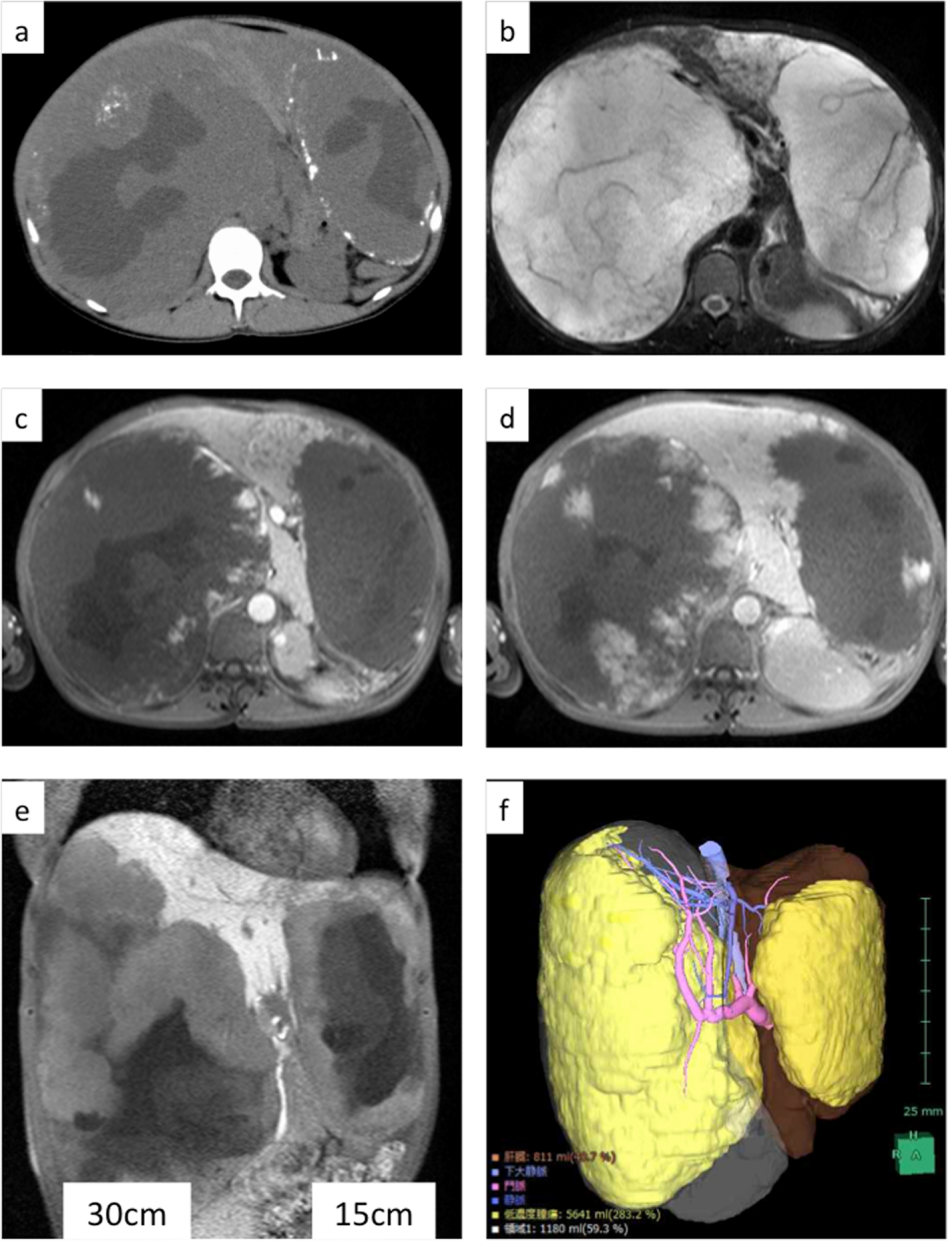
Plain computed tomography (CT) showed giant liver hemangiomas with lipiodol deposits (a). Magnetic resonance imaging (MRI) showed a 30-cm liver hemangioma in the right lobe and 15-cm liver hemangioma in the left lateral section (b T2 image, c arterial phase, d portal phase, e hepatobiliary image). There is normal liver parenchyma with tiny hemangioma in segment 4. According to 3DCT volumetry, the right liver volume was 60% (f)
